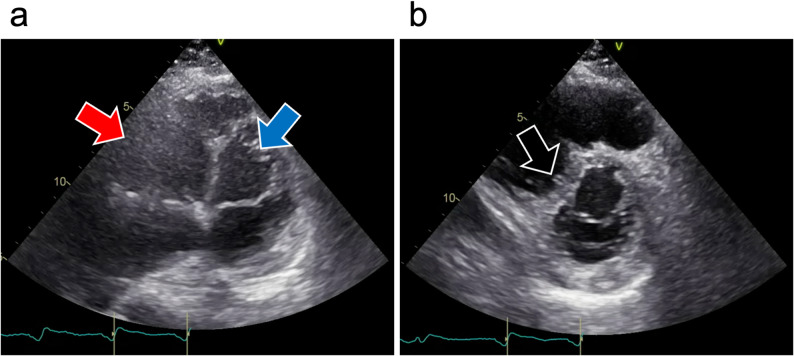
Preoperative transthoracic echocardiography. a: Apical four-chamber view demonstrating right ventricular dilation (red arrow) relative to the left ventricle (blue arrow). b: Left ventricular short-axis view at the mitral valve level showing flattening of the interventricular septum (black arrow)
